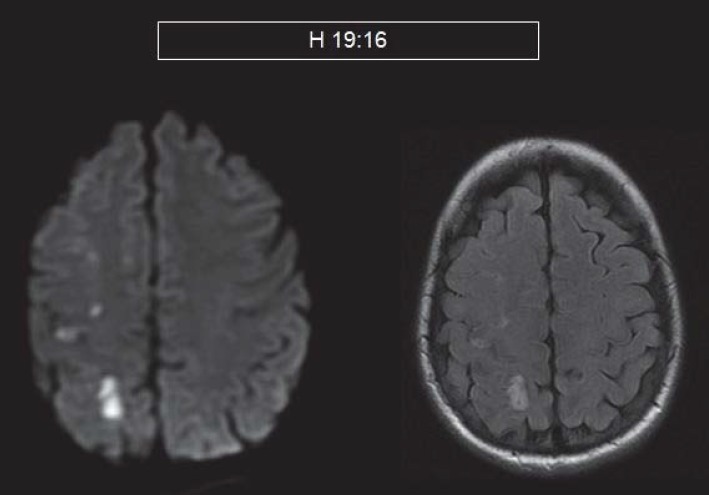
MRI- diffusion (left) and FLAIR hyperintensities (right) that suggests that there are irreversible ischemic lesions
